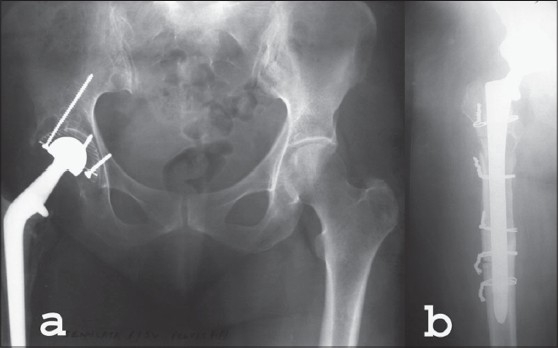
(a) Preoperative X-Ray (AP view) of a patient operated 20 years ago with painful loose cemented right THA. (b) Postoperative X-Ray (AP) of right hip of the same patient at four months of cementless revision stem (‘Solution’™)
